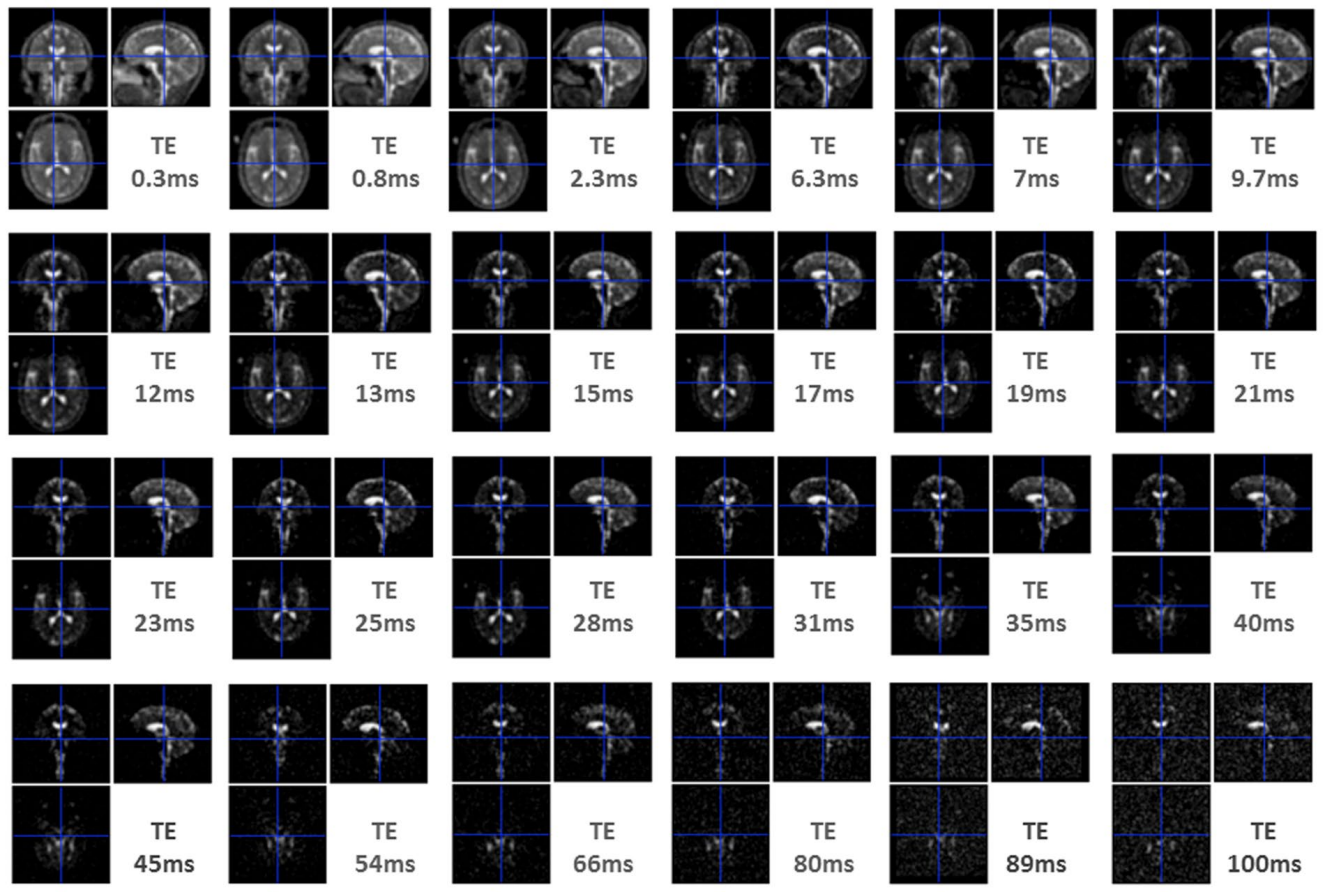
Exemplar ^23^Na-MRI images from a single subject across echo times. Note the decrease in signal as a function of the length of echo time (TE), and the change in the relative contributions from tissue and cerebrospinal fluid, with the latter providing most signal at long TEs.
